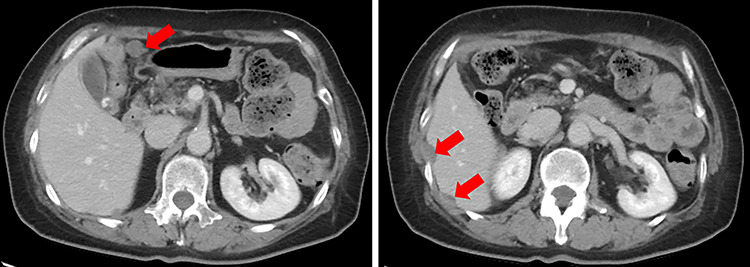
Enhanced CT. Numerous nodules were confirmed throughout the abdominal cavity (red arrows).
